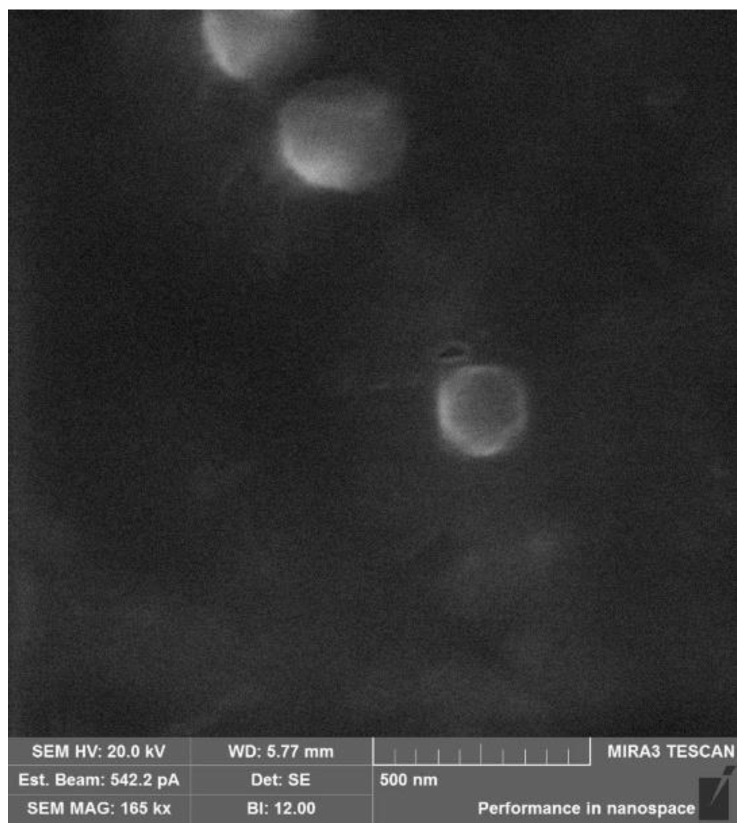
SEM images of the succinyl chitosan-colistin conjugates (SucCS-CT-10).
